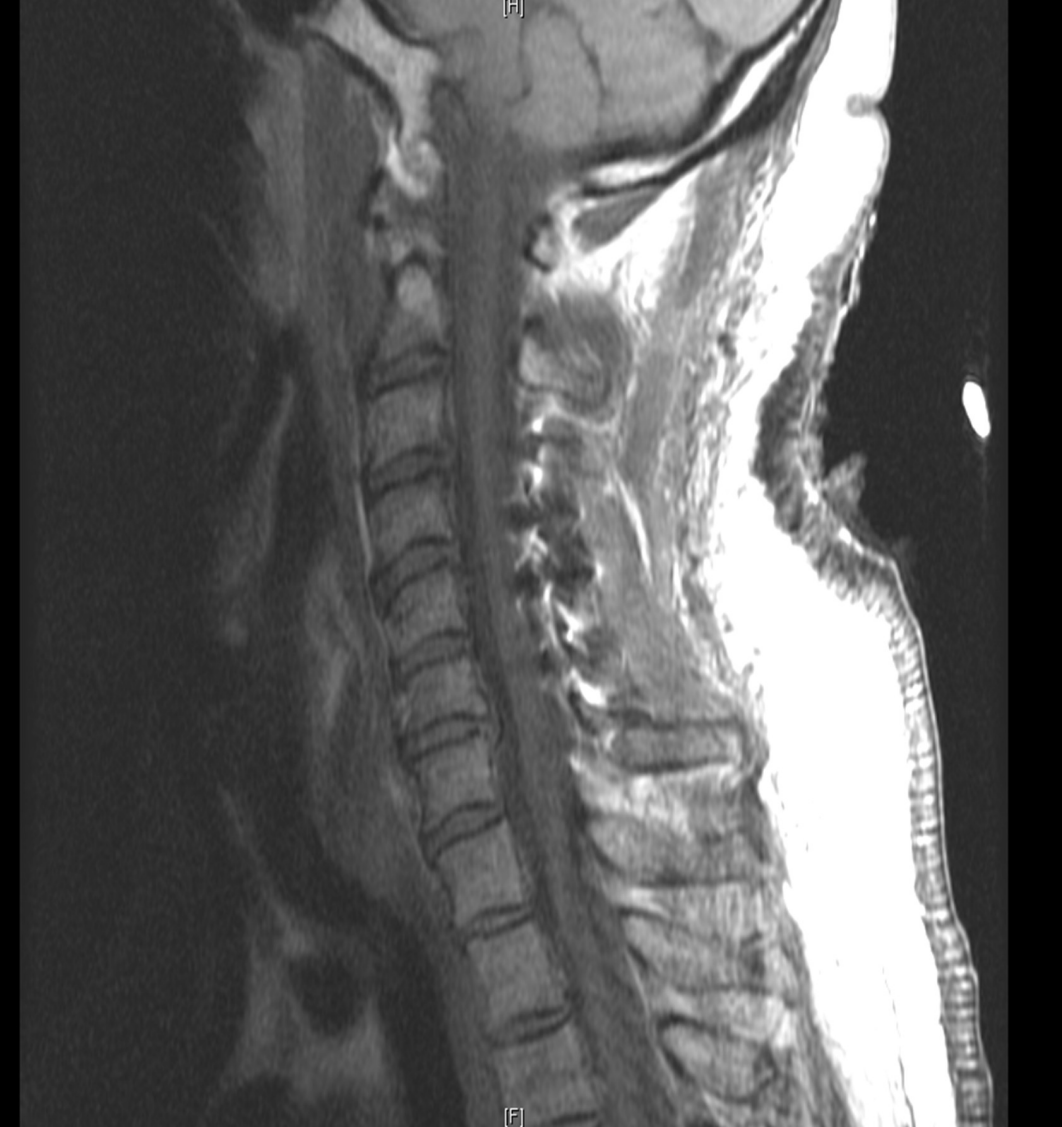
Normal post-operative sagittal T1-weighted MRI of patient's neck.
